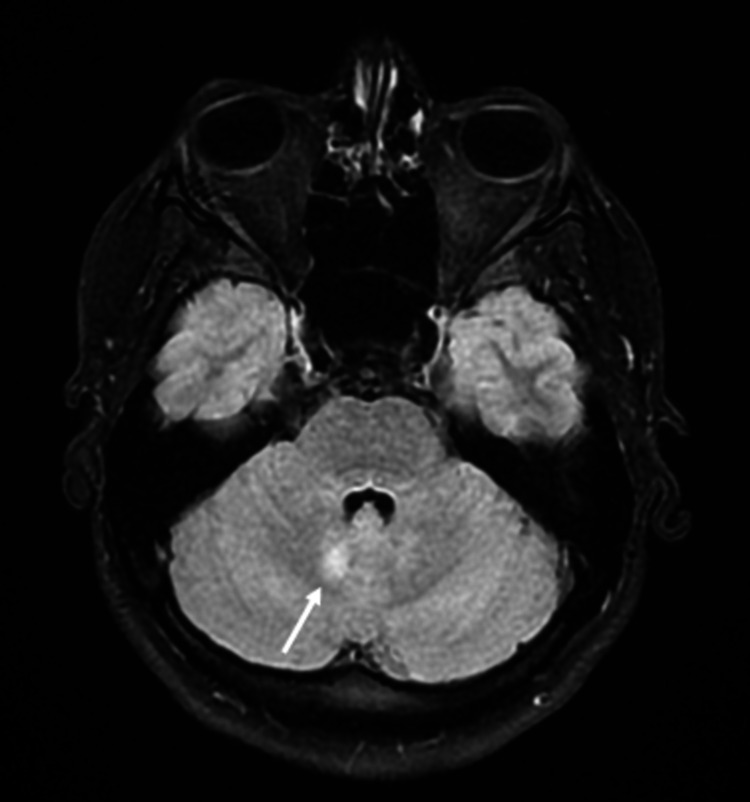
Brain MRI showing hyperintense FLAIR lesion in the cerebellar peduncle (arrow) MRI: magnetic resonance imaging; FLAIR: fluid-attenuated inversion recovery
